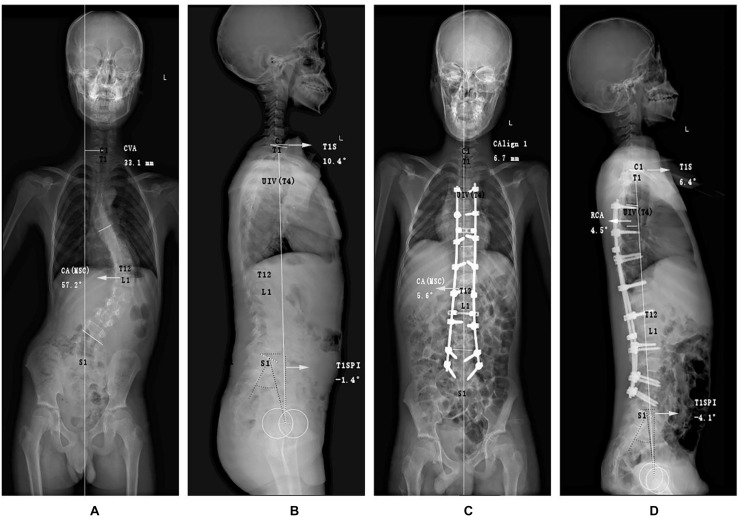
Graphic representations of special angles of an adolescent idiopathic scoliosis patient without PJK postoperatively. Different from the conventional measurements, the (A) anteroposterior and (B) lateral preoperative radiographs purposely included the following measurements for demonstrating coronal and sagittal malalignment: coronal vertical axis (CVA), the main curve coronal angle (CAMSC), T1 Slope (T1S), and T1SpinoPelvic inclination (T1SPI). At immediate postoperative X-ray films (C,D), rod curve angle (RCA) was also measured. PJK, proximal junctional kyphosis.
